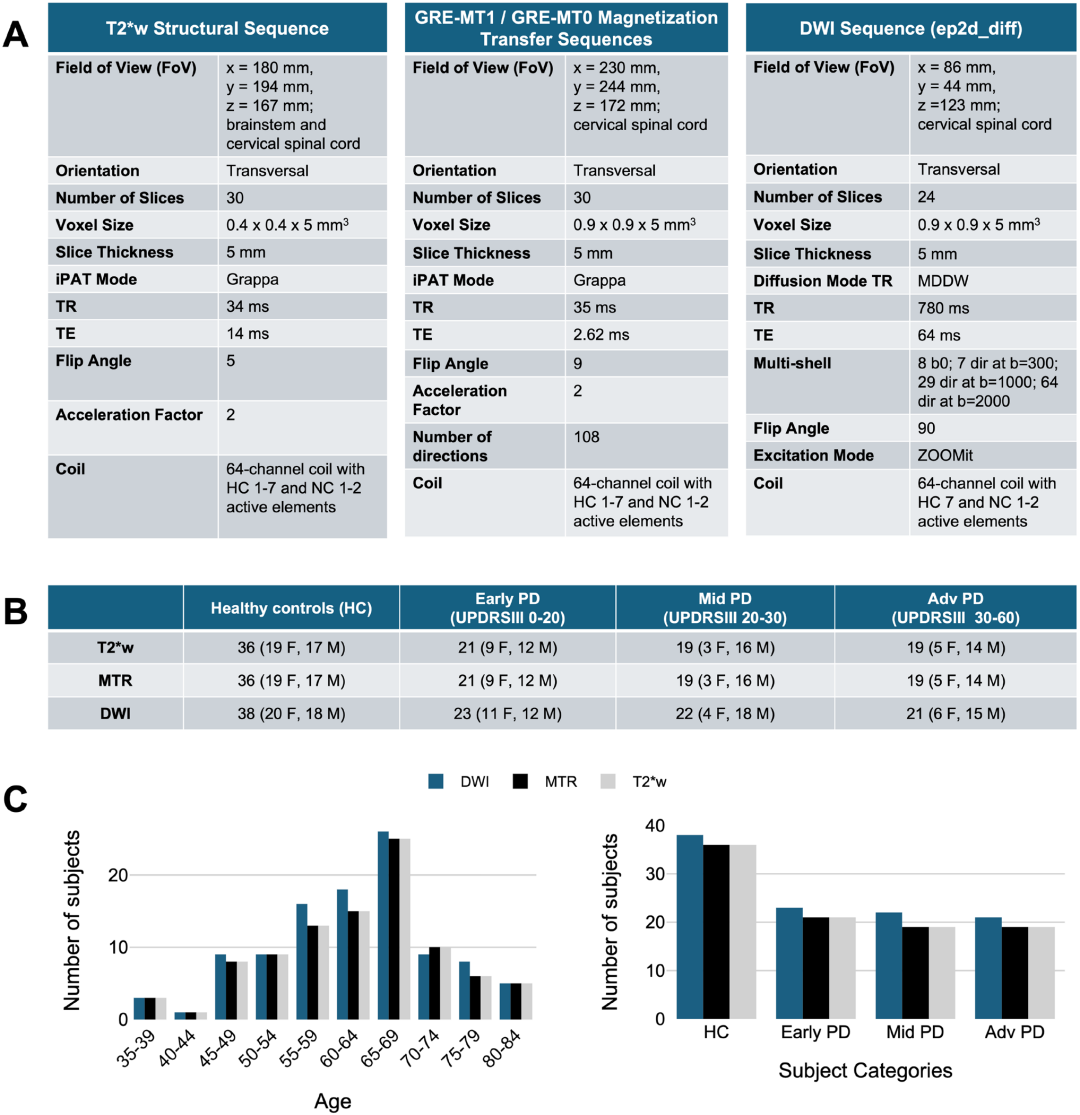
(A) Acquisition parameters for T2*w, MTR, and DWI; (B) Number of participants per category (controls, early PD, mid PD and advanced PD) for each sequence according to their UPDRSIII score. (C) Age and UPDRSIII distributions.
